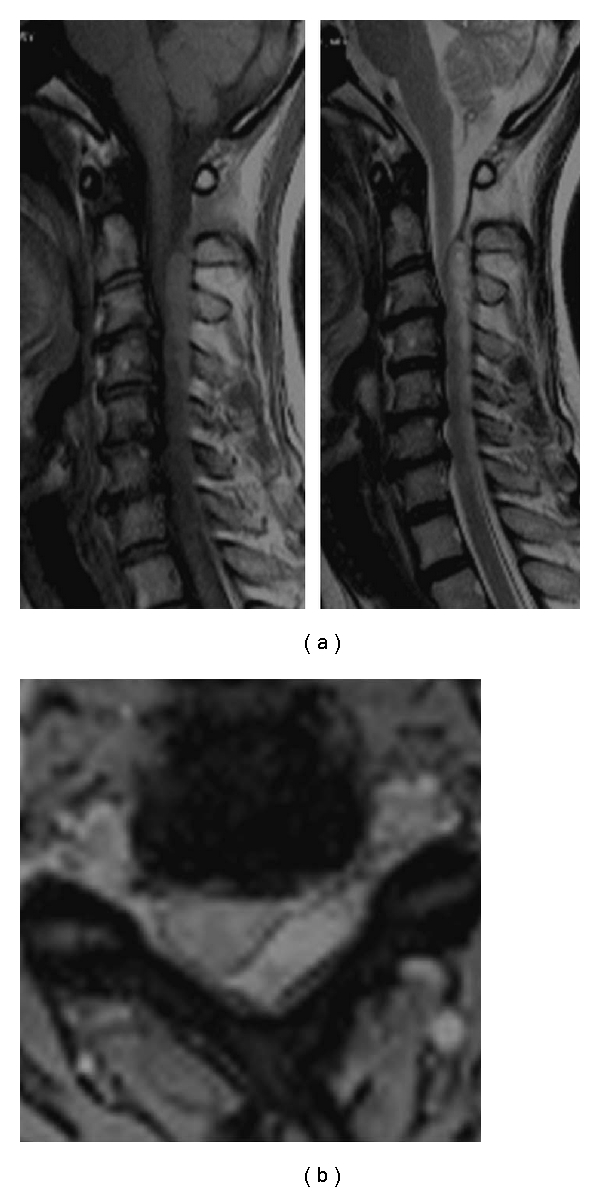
Initial sagittal (a) and axial (b) MR images showing a left posterolateral epidural hematoma at the C2-C4 level with spinal cord compression.
